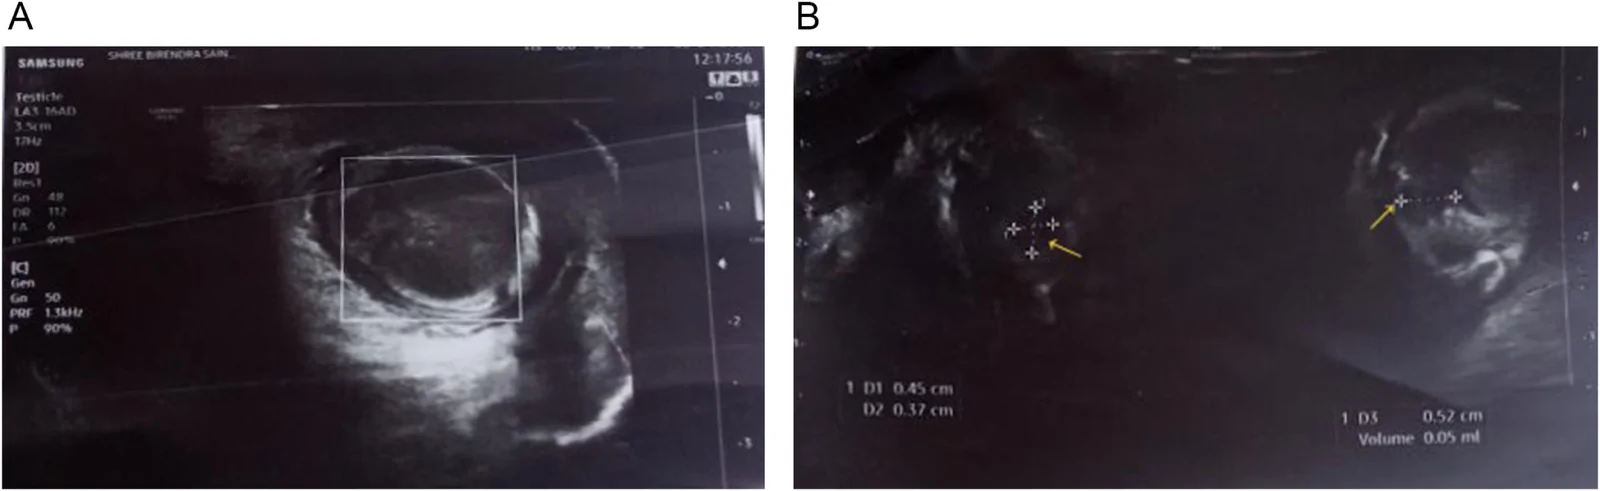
(A, B) Scrotal ultrasound demonstrating a testicular fracture with a loculated collection and no detectable vascularity in the left testis.
